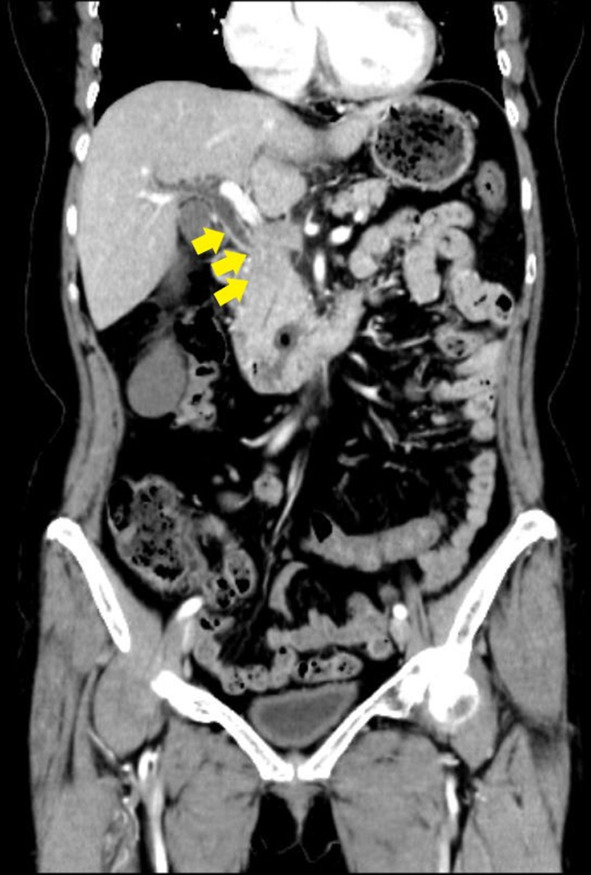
CECT scan of the abdomen showed thickening of the walls of the middle to distal bile ducts (arrows) and dilated intrahepatic bile ducts. No mass was evident around the bile duct wall. CECT, contrast‐enhanced computed tomography.
